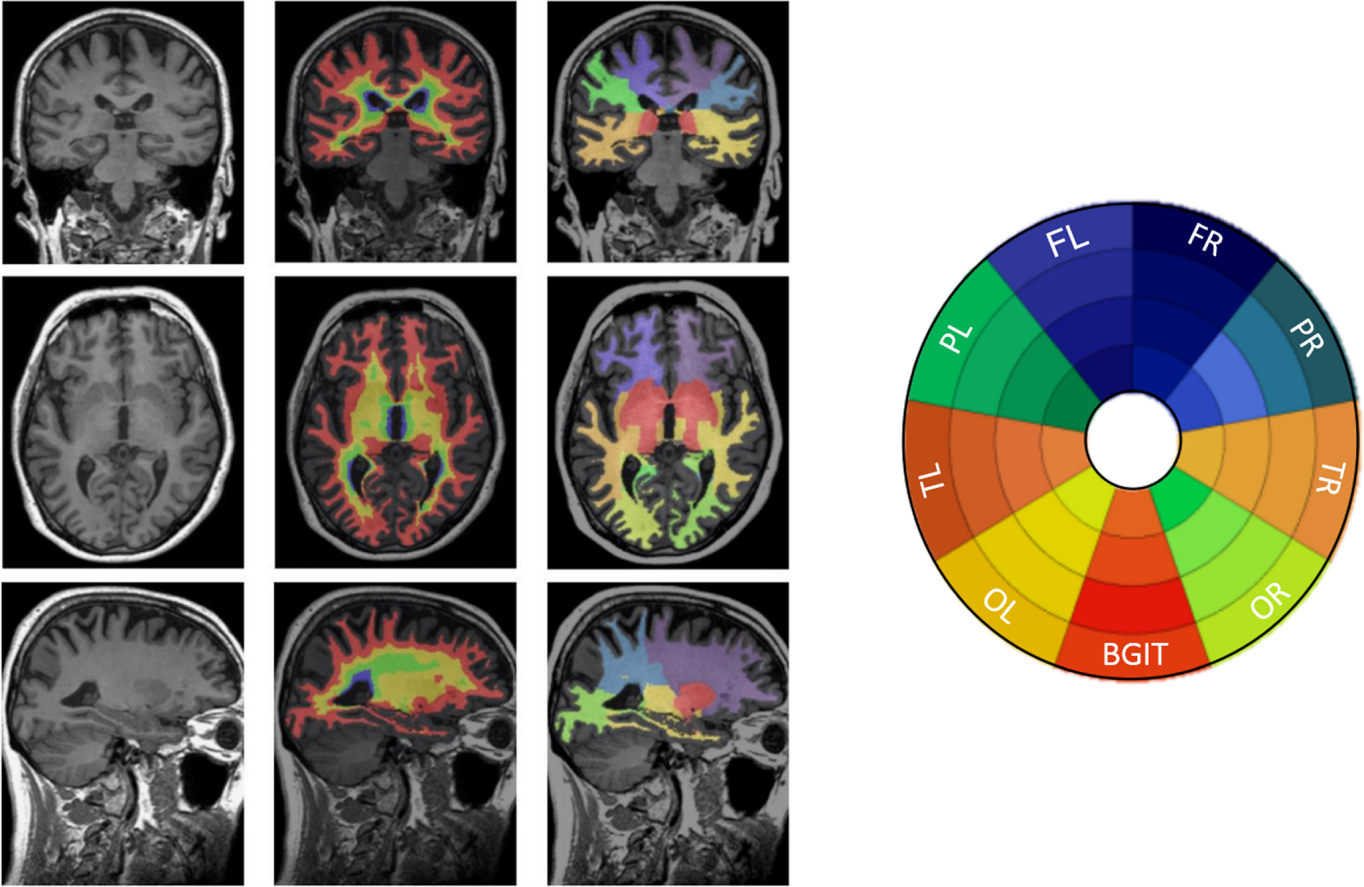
Example of the segmentation of one participant and the Bullseyes plot representation. On the left figure, the left column, T1w MR image from one participant is presented in the three views: coronal, axial and sagittal (from up to bottom). In the central and right columns, the same views are shown with the layer and lobar segmentations overlaid, respectively*.* Abbreviations: FR, frontal right; FL, frontal left; TR, temporal right; TL: temporal left; PR, parietal right; PL, parietal left; OR, occipital right; OL, occipital left and BG, basal ganglia
